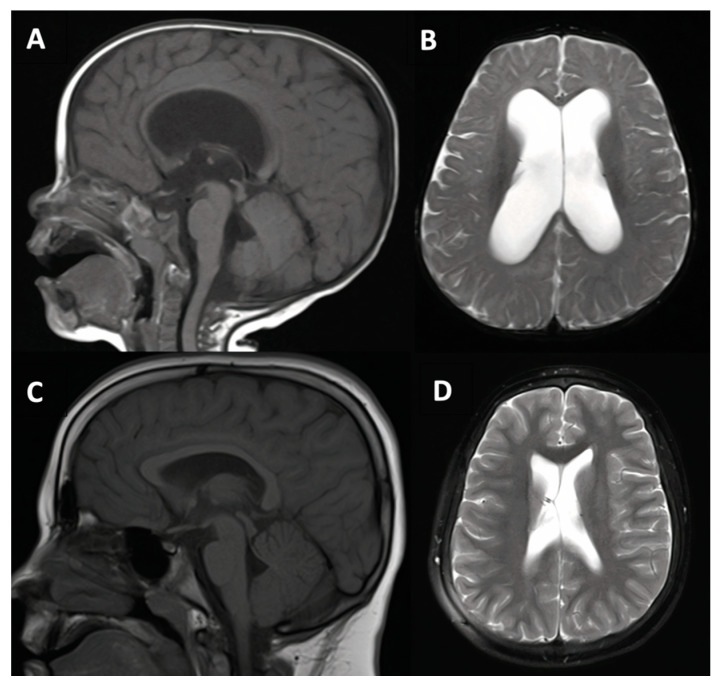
18-month-old female patient with premature birth and developmental delay (case #4). Pre-operative (A,B) and 8 years post-operative (C,D) T1-weighted mid-sagittal and T2-weighted axial magnetic resonance imaging (MRI).
